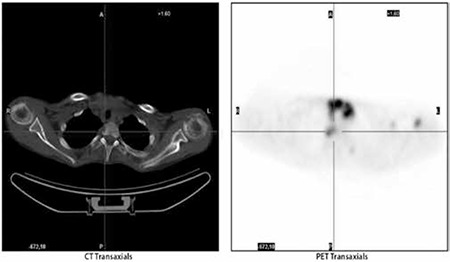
Axial PET part of the PET/CT study showing the FDG accumulation corresponding to the soft tissue involvement in the right side of the T2 vertebral body
